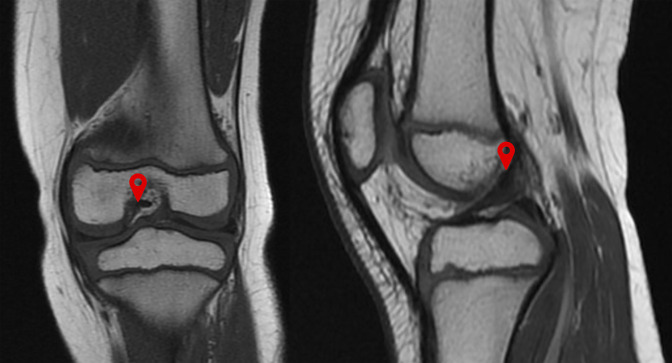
Coronal and sagittal T1-weighted MRIs showing the knee of a 6-year-old girl, marking the femoral insertion of the anterior cruciate ligament.
